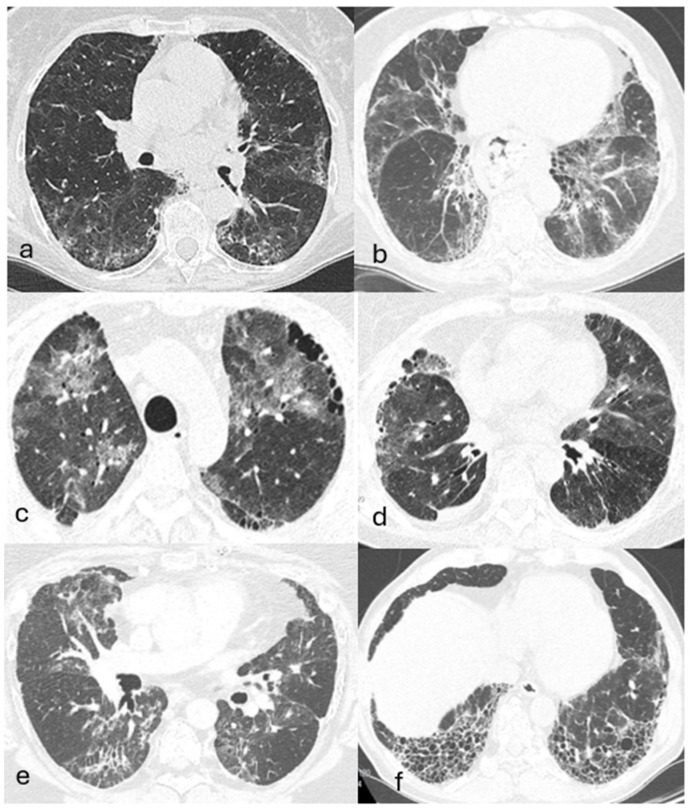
(a,b) HRCT scans show an NSIP pattern in the course of isolated ANCA-MPO-ILD. Bilateral ground–glass opacities, reticulation, and traction bronchiectasis are visible, with typical subpleural sparing and preserved volume in the lower lobes. Small subpleural honeycomb-like areas are also visible, which, although rare, can be observed in NSIP. (c,d) Bilateral interstitial lung changes in MPO-AAV with ground-glass and linear band-like opacities. Small subpleural honeycombing areas are also visible, along with volume loss in the right lower lobe and pleural thickening on the right side. (e) ILD in the course of PR3-AAV. Scan shows bilateral ground-glass opacities, band-like reticular abnormalities, and traction bronchiectasis, without evidence of honeycombing. (f) Bilateral honeycombing areas predominantly involving the lower lung fields, consistent with a UIP pattern.
